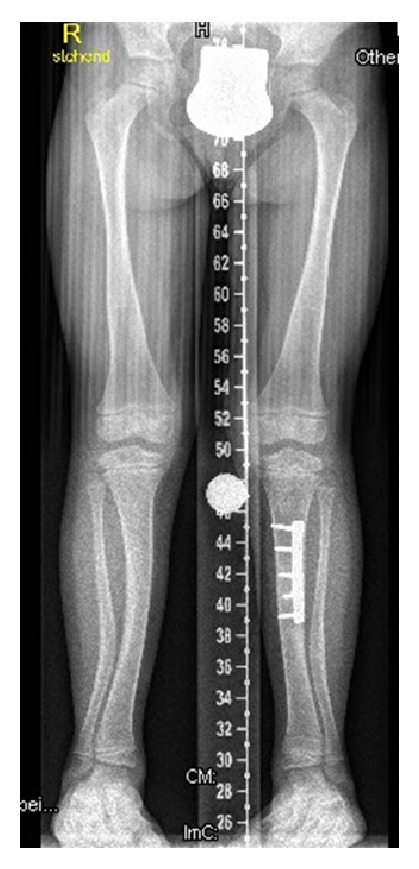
AP standing lower limb radiograph at the age of 11 years (after operation) showed lessening and improvement of the lower limbs deformity following the closing-wedge osteotomy of the left tibia and fibular-osteotomy.
